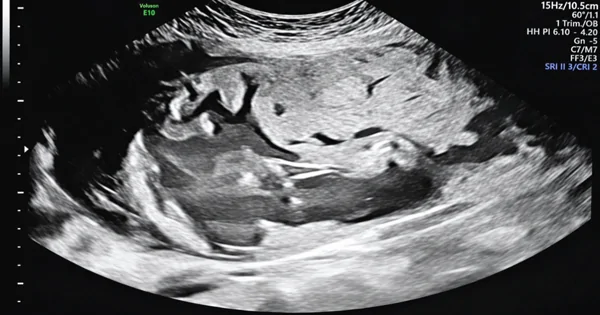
Ultrasound features suspicious for placenta accreta spectrum. Gray‐scale ultrasound demonstrates an abnormally thickened low‐lying anterior placenta with multiple placental lacunae and an intraplacental hematoma.
